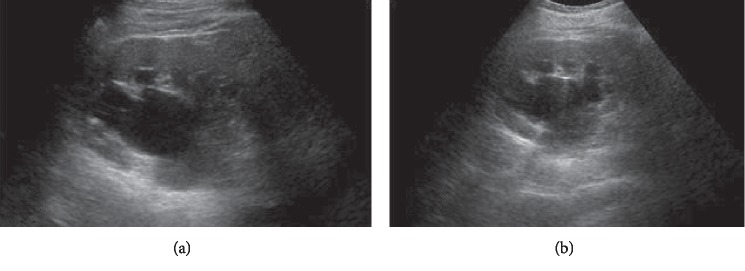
Serial ultrasound images of the right kidney done at 6 month intervals after diagnosis of the kidney obstruction. Showing similar ureteral and pelvicalyceal dilation (a and b) over the span of 6 months with no change in the renal cortex thickness.
